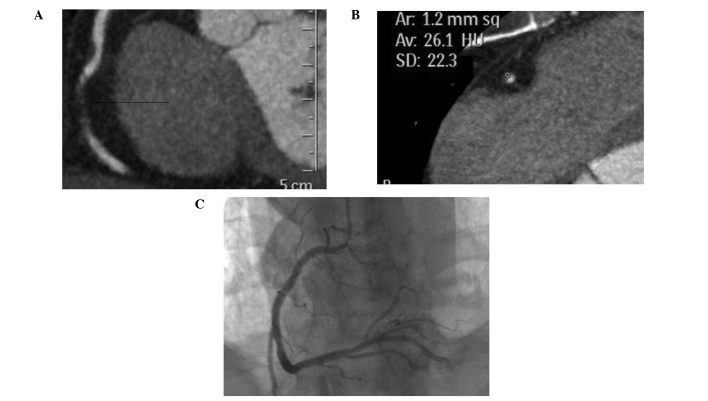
A 57-year-old female with an 11-year history of T2DM. (A and B) Soft plaques in the middle segment of the right coronary artery with an eccentric and smooth surface (black arrow). The CT value was 26 Hu. (C) The plaque was identified through routine coronary angiography. CT, computed tomography; T2DM, type 2 diabetes mellitus.
